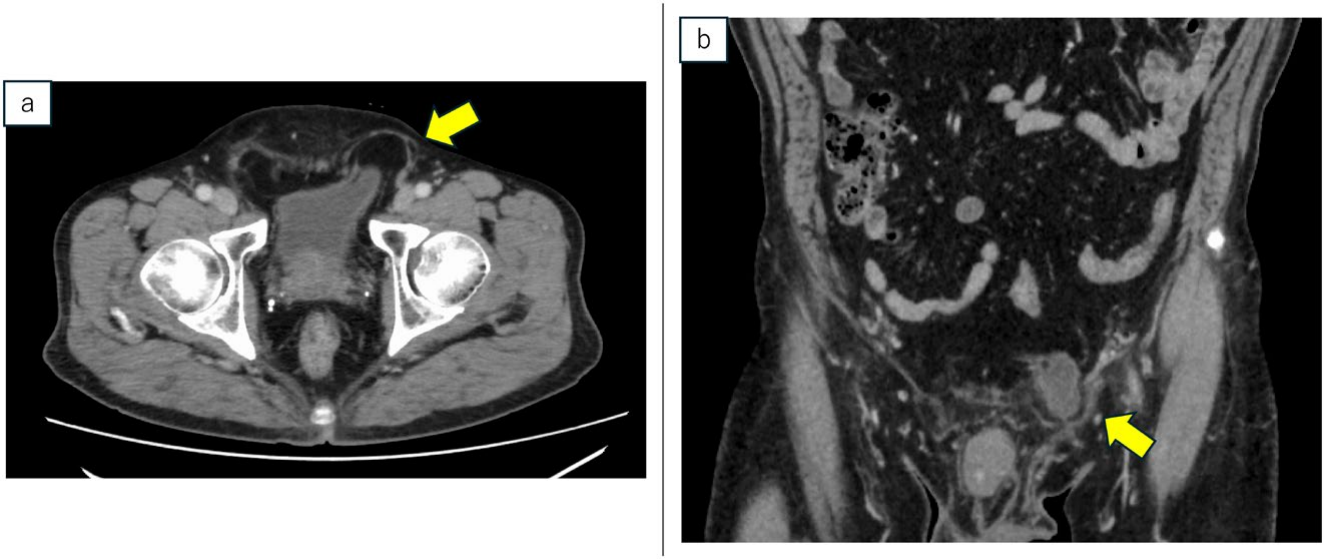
(a) Axial and (b) coronal computed tomography scan revealed left direct bladder hernia with a part of the bladder protrusion.
